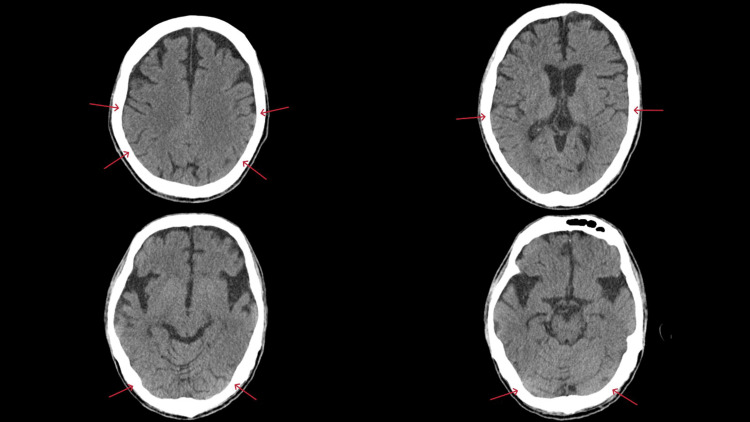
CT scan images after treatment show complete resolution of brain edema Compared to the CT scan taken at the time of arrival, the sulci are clearly visible (red arrows), suggesting improvement of brain edema.
